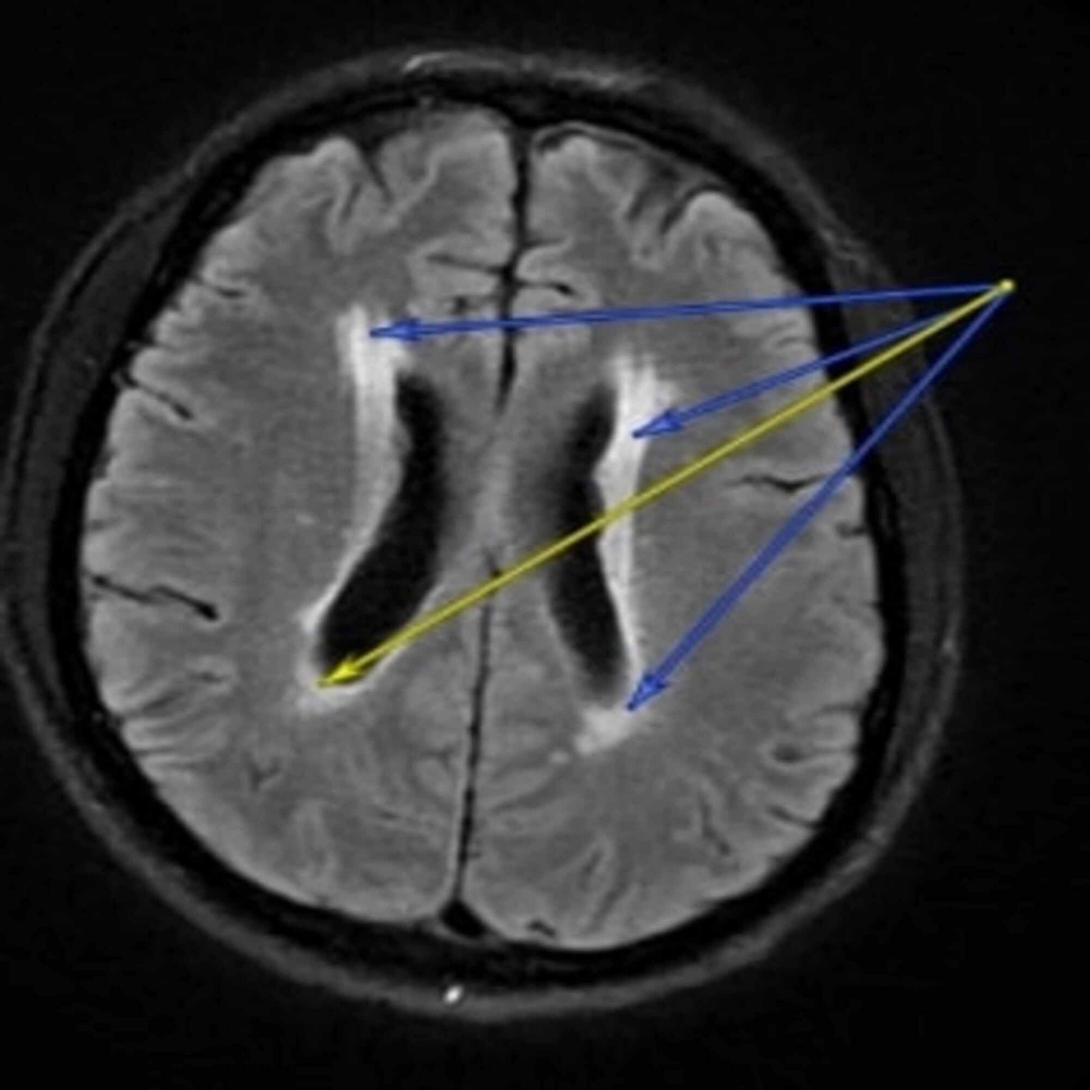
MRI brain with periventricular white matter hyper-intensities. Both yellow and blue arrows showing periventricular hyper-intensity seen on MRI
